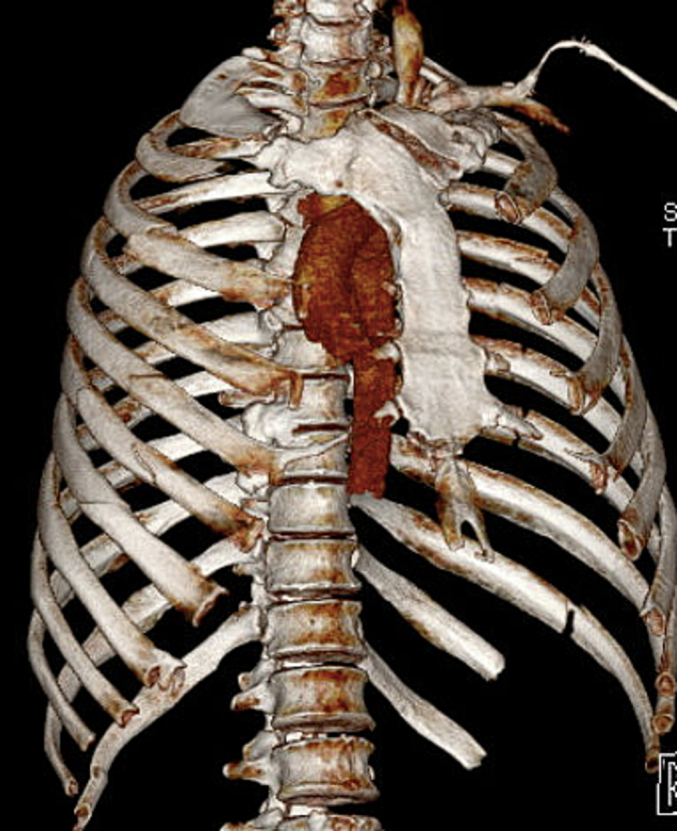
Preoperative CT chest with 3D Reconstruction demonstrating right-sided rib fractures.
